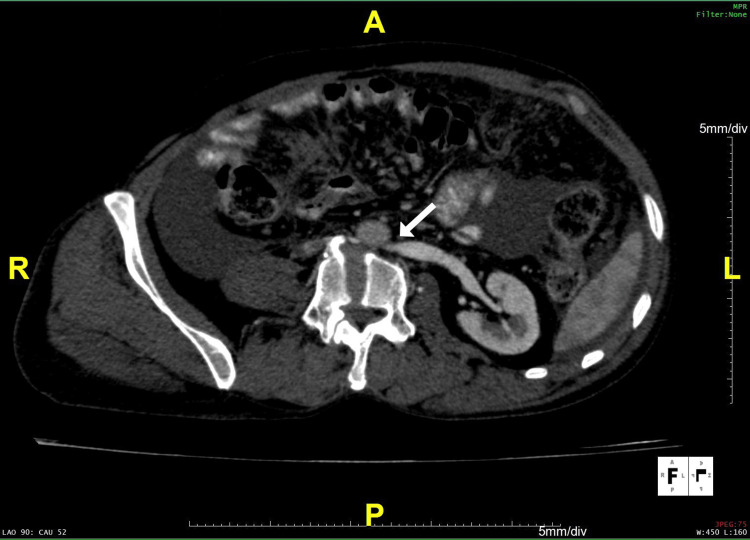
Axial section on contrast-enhanced CT with multi-planar reformation, showing the compression of the left renal vein between the abdominal aorta and vertebral body
